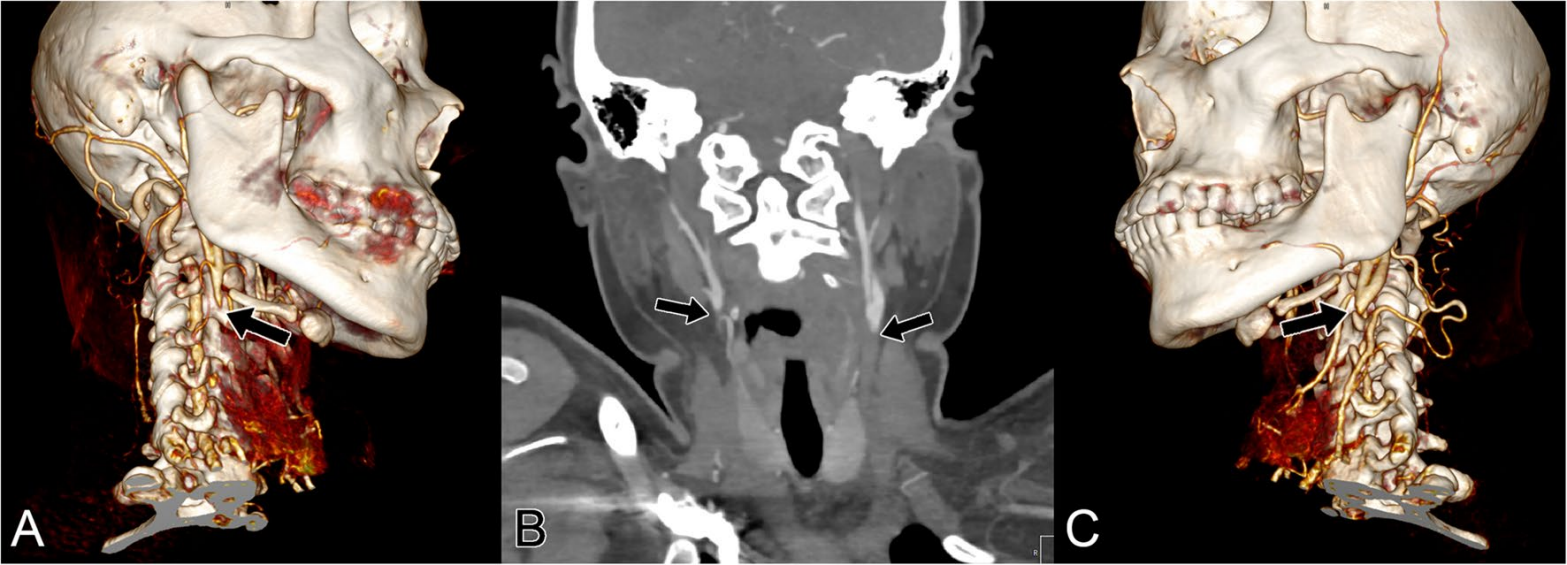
Neck CTA showing bilateral CCA occlusion (black arrows). Figure 3A and 3C: CTA 3D reconstruction. Figure 3B: MIP imaging modality
